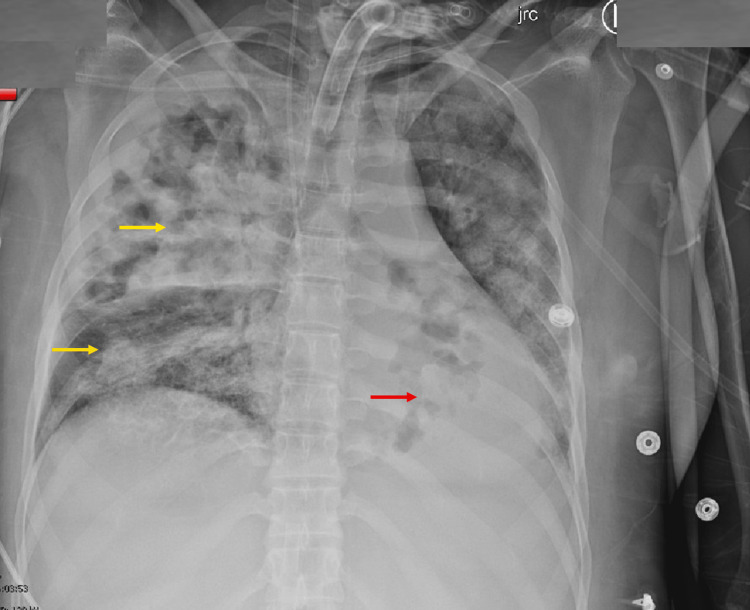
Anterior-Posterior Chest Radiograph on Presentation The chest radiograph demonstrates a dense cavitary right upper lobe pneumonia (yellow arrow), increasing opacities in the right lower lobe (orange arrow), and opacities and dense left lower lobe consolidation (red arrow).
